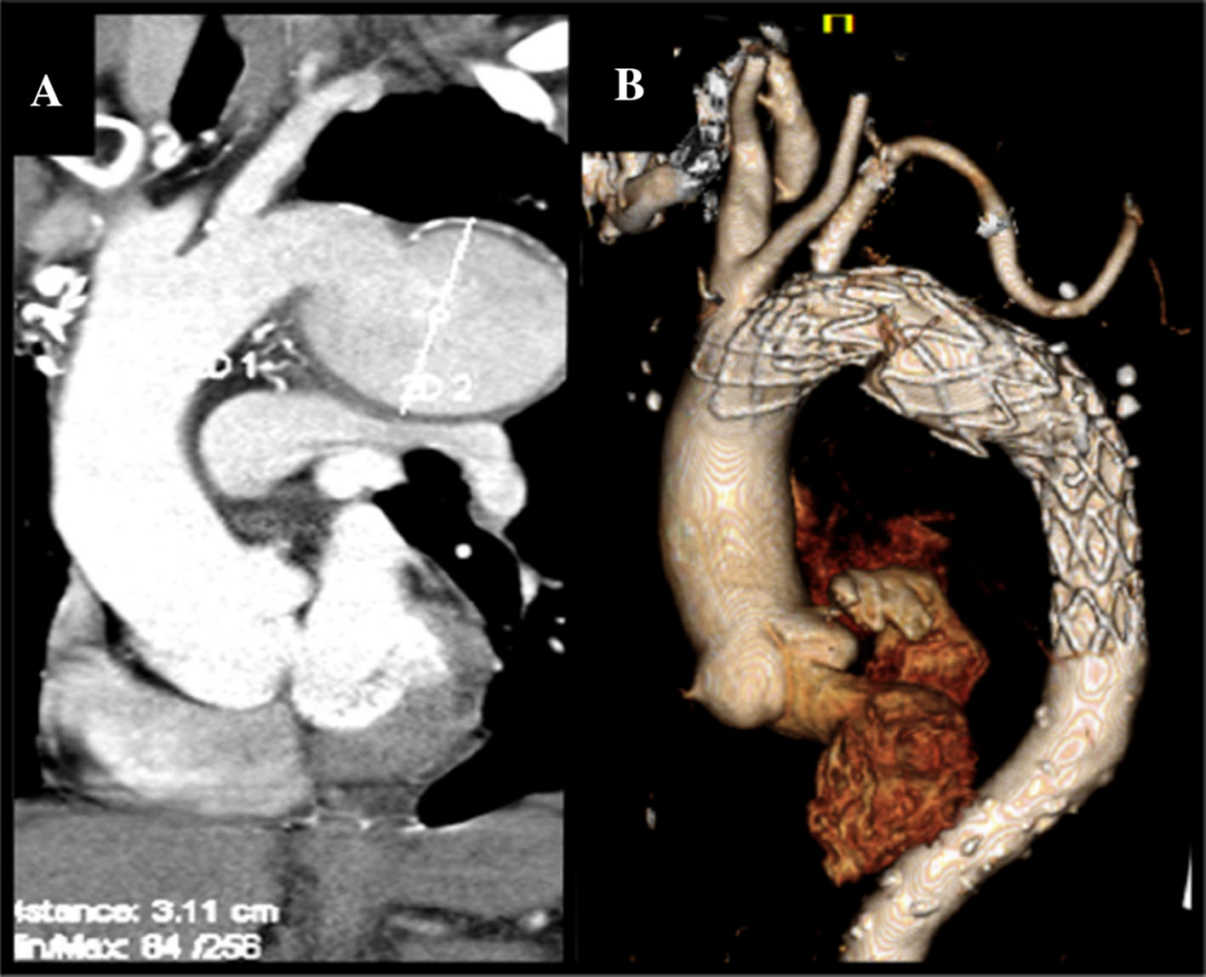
Aortic CT of the first patient before (A) and 6 months after the fenestrated TEVAR (B). Patency of the LSA stent is maintained after 6 months. No endoleak was observed
